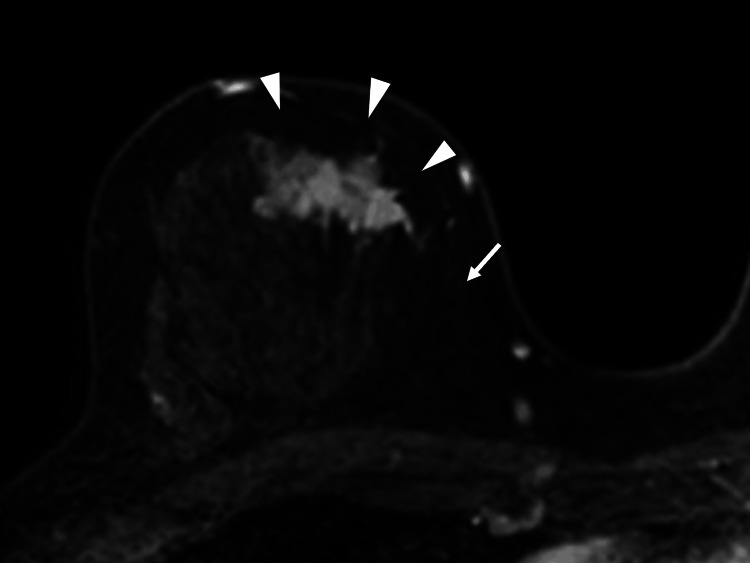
Contrast-enhanced magnetic resonance imaging (axial section): tumor-catheter distance measurement Similar to the computed tomography findings, an irregularly enhancing mass is seen in the right breast (arrowheads), with the shunt catheter located on the medial side (arrow). The medial edge of the tumor is approximately 20 mm from the catheter.
